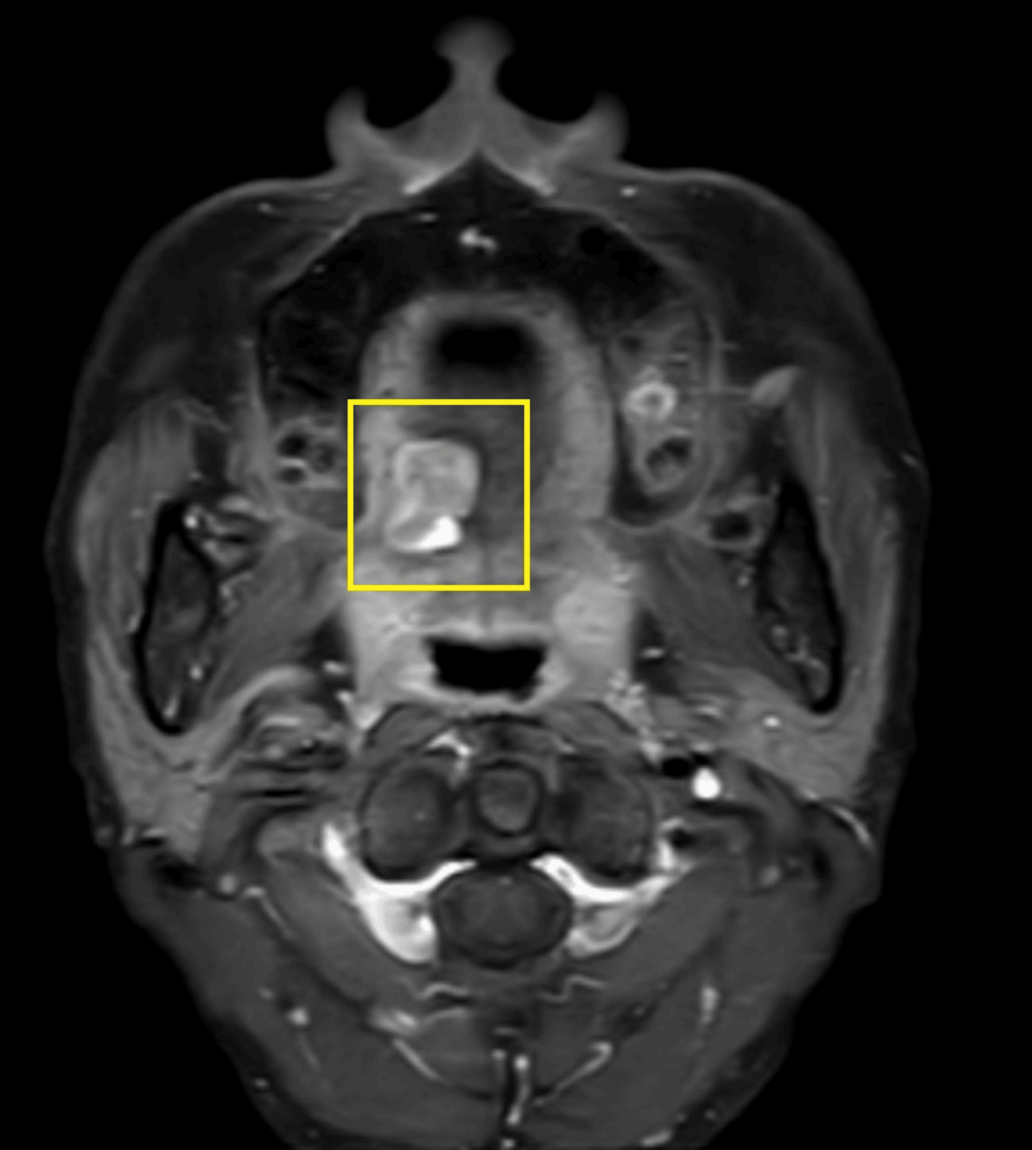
MRI of the oral cavity (axial view) MRI: magnetic resonance imaging
